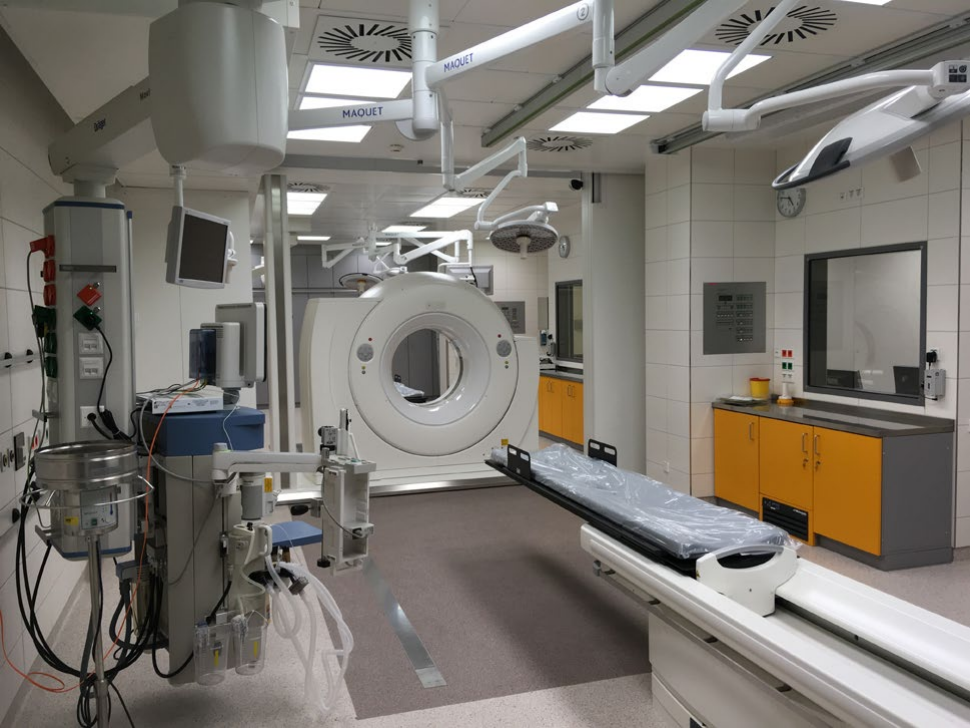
Large-scale trauma resuscitation area with a dual-room sliding gantry CT scanner
